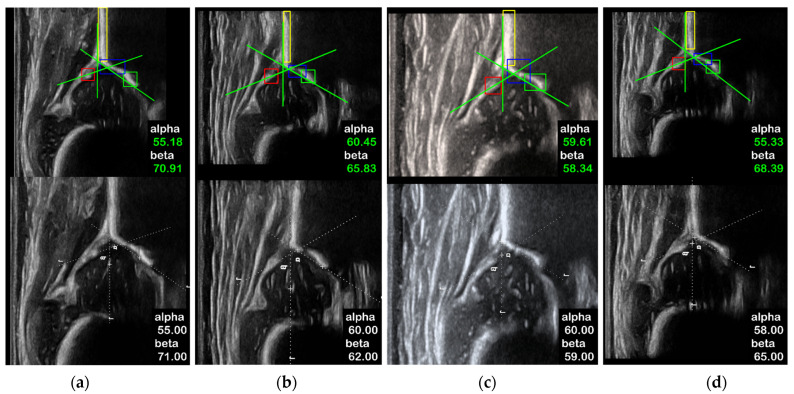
Qualitative comparisons on the angle measurement between the DM w. SPSM (upper) and sonographers (lower). The standard plane frames selected from the same US video are usually different, but the planes selected by DM w. SPSM and the angle measurement results are also reliable. The absolute differences between DM w. SPSM and sonographers are (a) Δα = 0.18°, Δβ = 0.19°; (b) Δα = 0.45°, Δβ = 3.83°; (c) Δα = 0.39°, Δβ = 0.64°; (d) Δα = 2.67°, Δβ = 3.39°.
